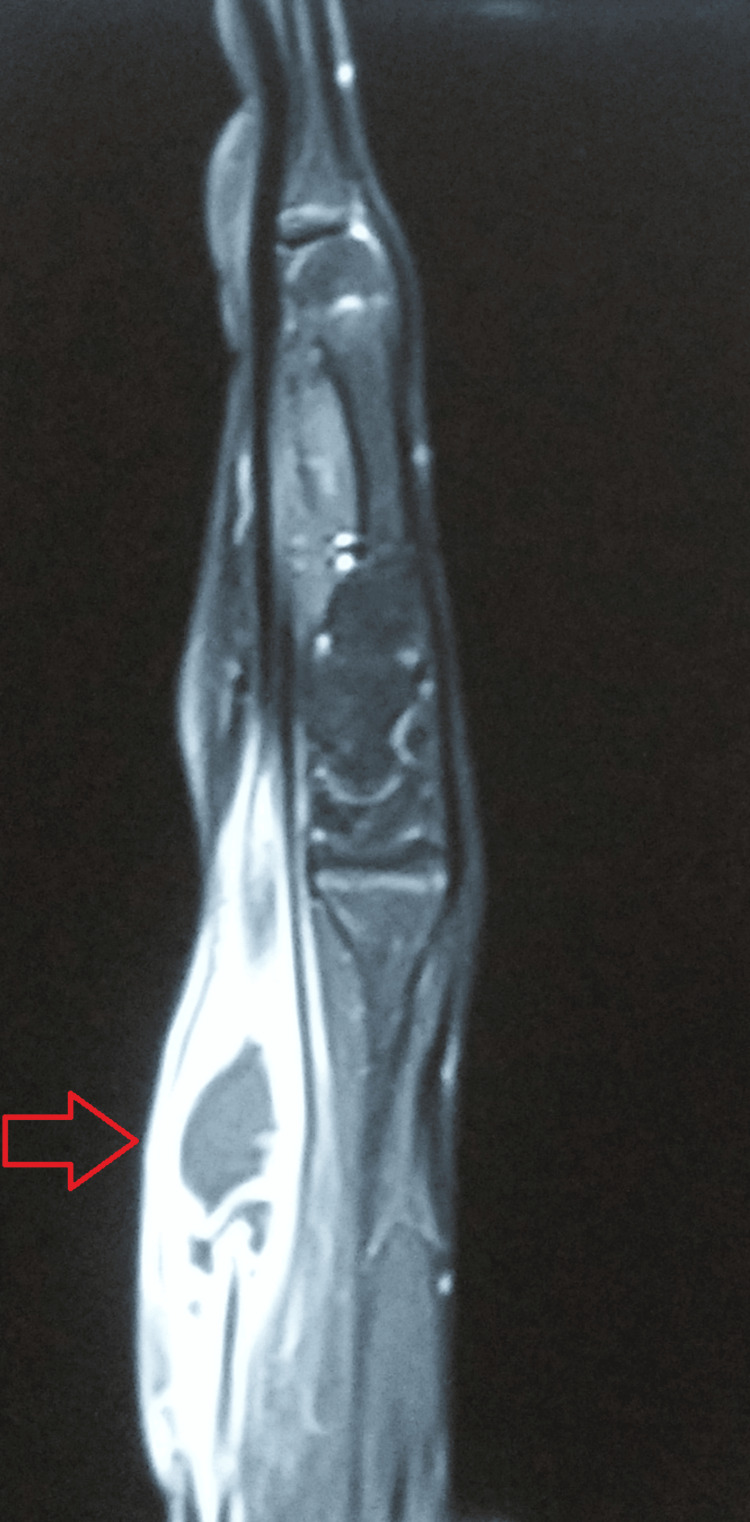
CEMRI showing peripherally enhanced collection in the distal forearm in the volar aspect with significant soft tissue edema. CEMRI: Contrast-enhanced magnetic resonance imaging
